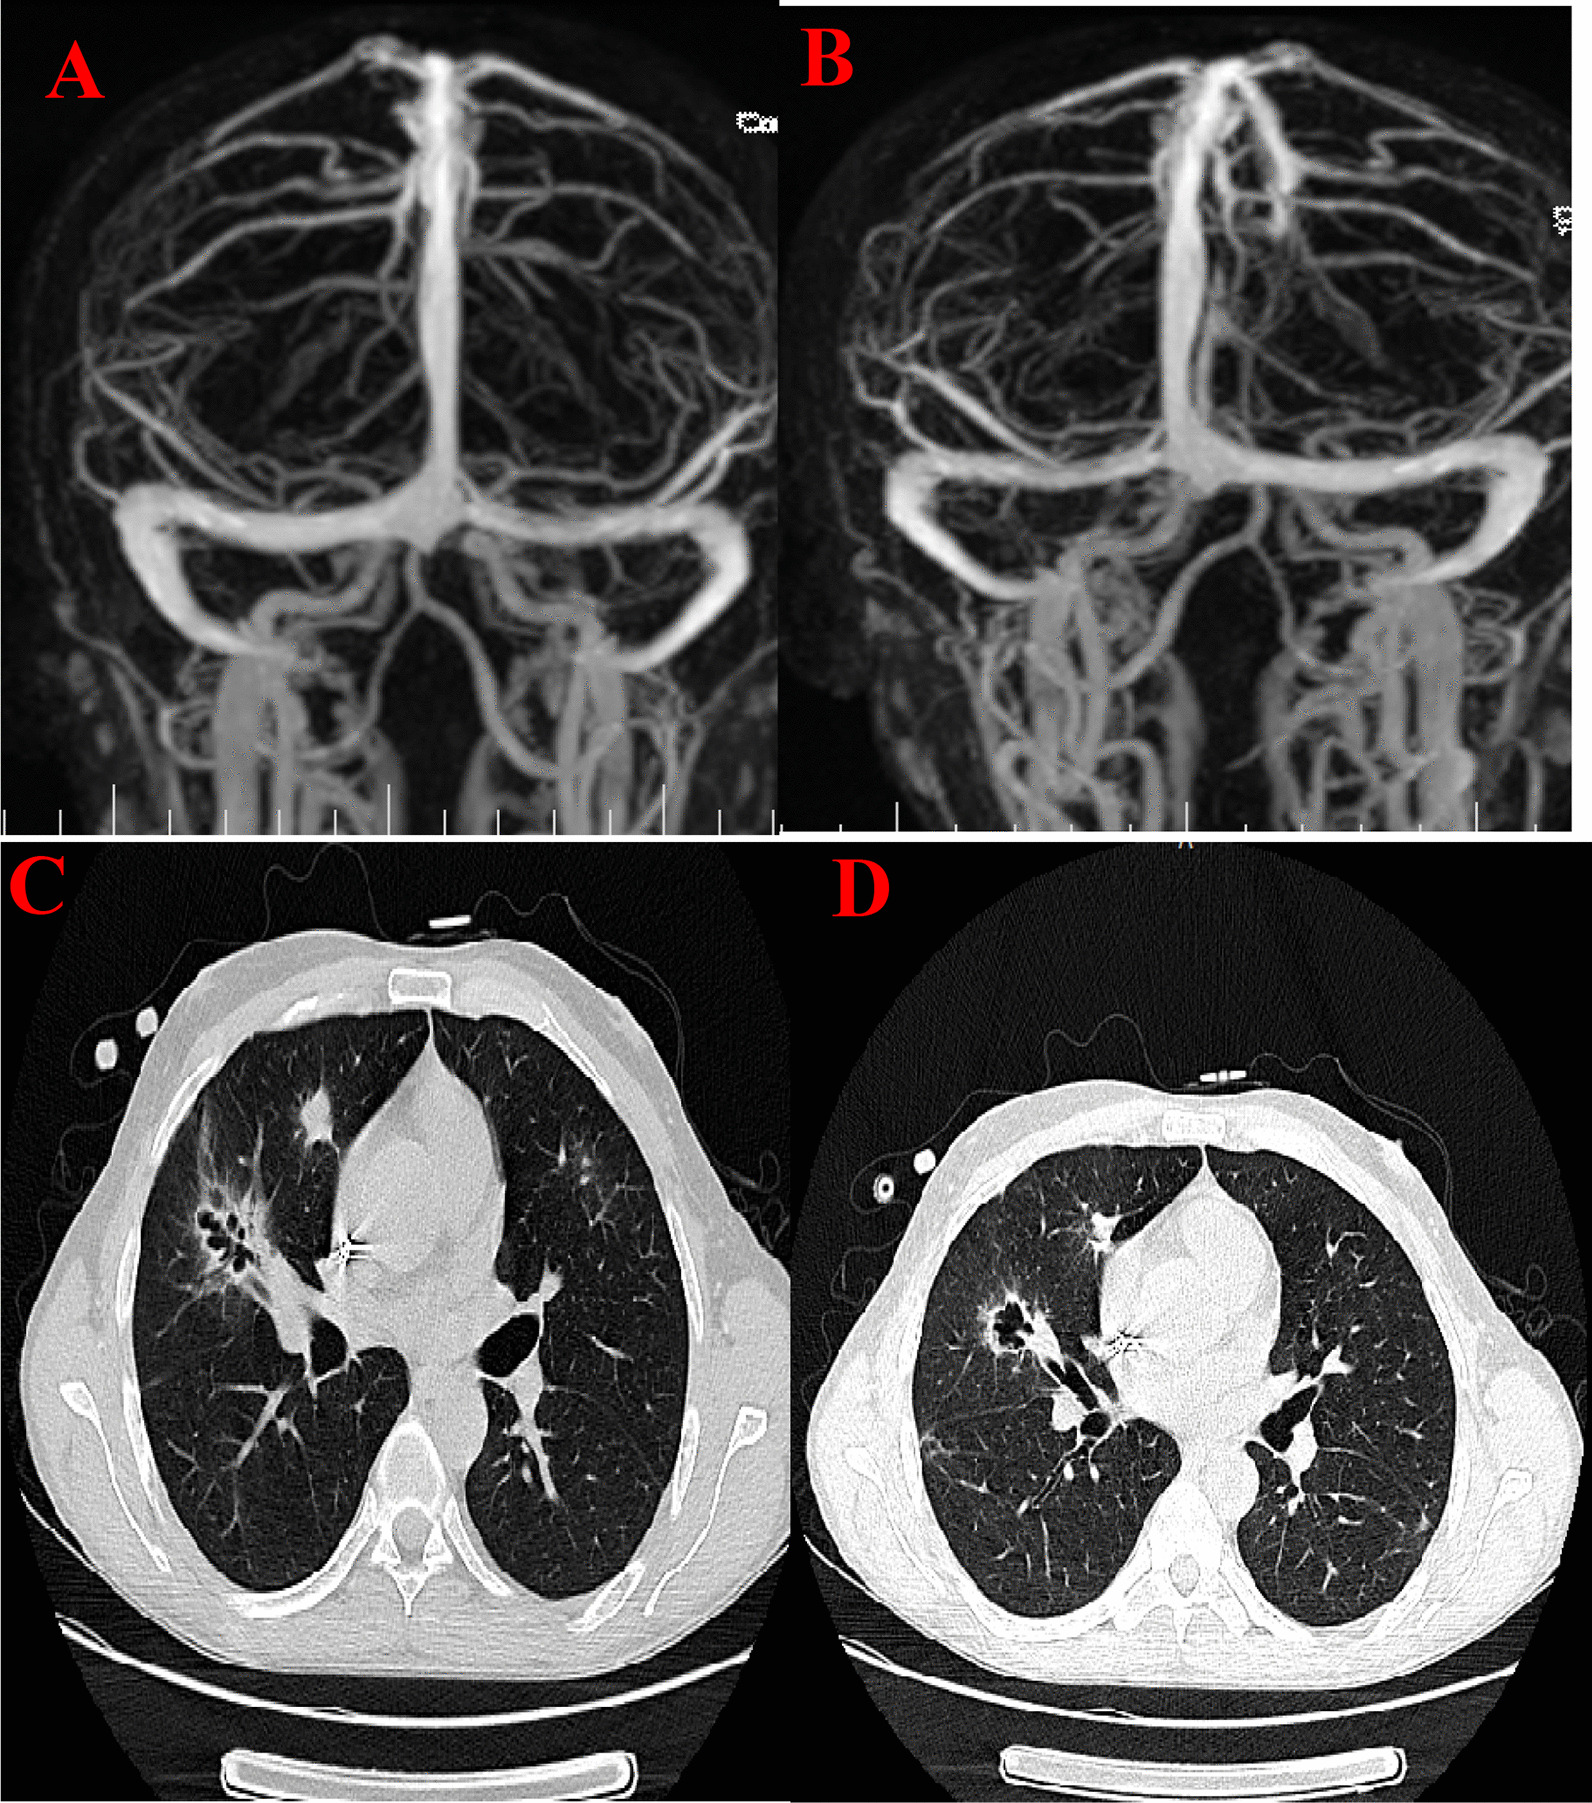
Magnetic resonance venography imaging after a 6 months treatment with warfarin (A and B): no evidence of thrombosis in the transverse and sigmoid sinus can be noted. CT image (C and D): multiple cavitary lesions and ground glass opacities in both lungs
